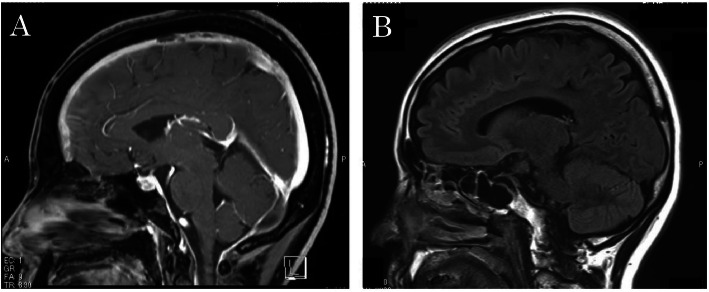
a Sagittal T1-weighted gadolinium-enhanced MRI. Baseline imaging shows diffuse pachymeningeal enhancement, sagging of the brain, enlargement of the pituitary gland, and coarctation of the lateral ventricles. b Imaging after EBP placement shows resolution of brain sagging, smaller pituitary gland, resolution of pachymeningeal enhancement, and resolution of lateral ventricle narrowing. EBP, epidural blood patch; MRI, magnetic resonance imaging
